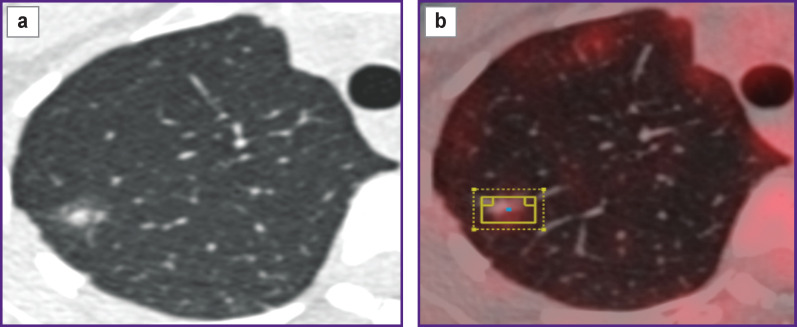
Diagnosis: “acinar adenocarcinoma of the upper lobe of the right lung; G2; pT1aN0cM0; IA”. The study was performed on a PET/CT Discovery 690: (a) CT scan in the S3 segment of the right lung detects a subsolid lesion with the total size 12 and 8 mm of the solid part; (b) PET detects a lesion that minimally accumulates radiopharmaceutical; SUV_max_=0.9; RC=0.27; SUV_correct_=3.5
